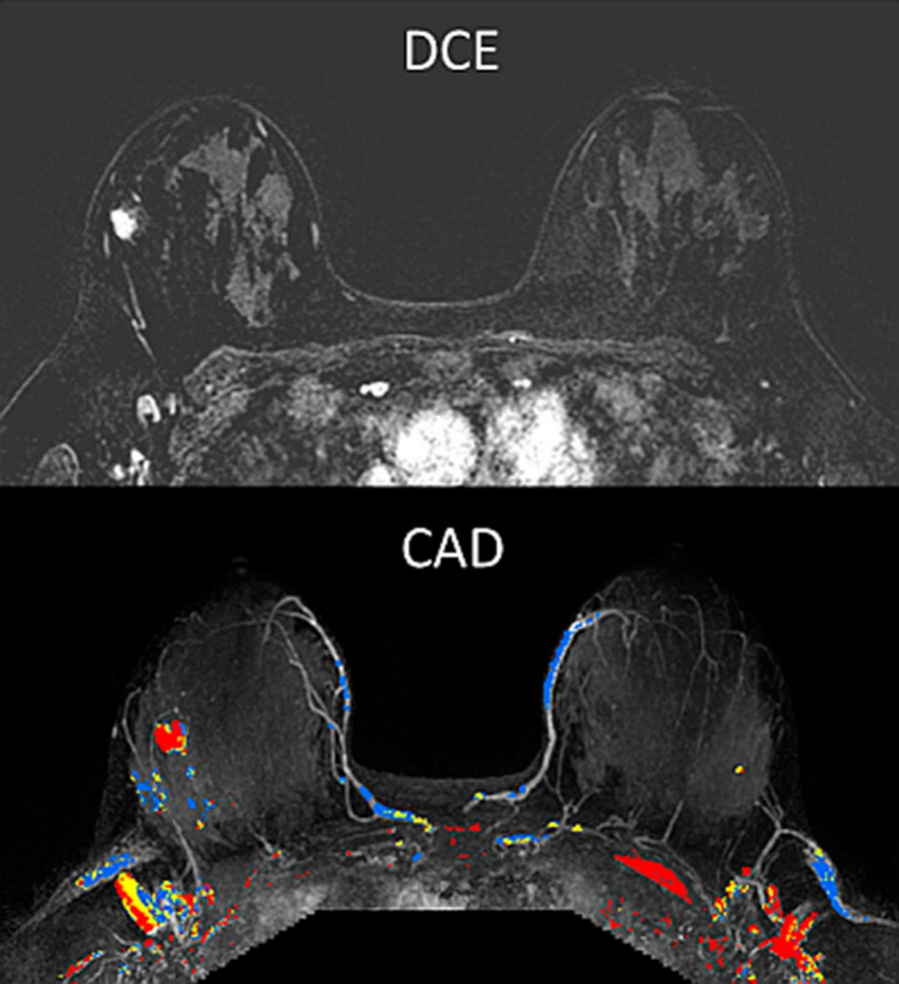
Preoperative MRI during lactation. Axial subtracted DCE-MRI and CAD images of a 37-year-old PABC patient, lactating for 3 months are shown. *Note*: DCE reveals the presence of a single small lesion in the right upper outer quadrant (12 mm IDC) with excellent conspicuity, comparing with the surrounding moderate BPE. The tumor exhibited the suspicious wash-out pattern (red) on CAD MIP image, while slight BPE exhibited persistence benign wash-in pattern (blue)
